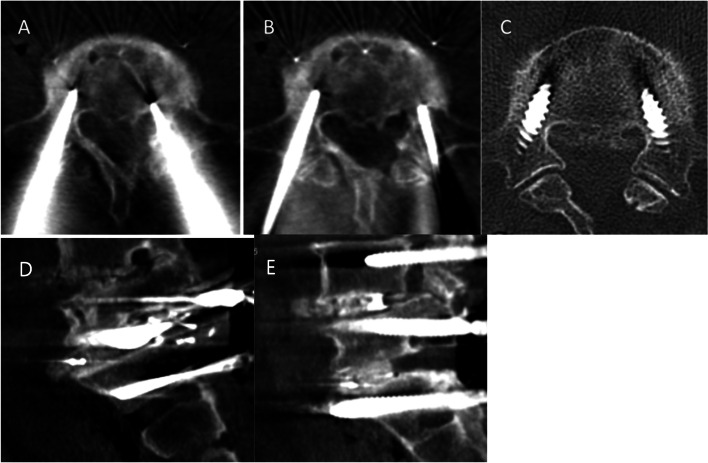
Examples of intraoperative computerized tomography (CT) images. A Initial intraoperative image demonstrating a medial misplacement of guiding needle in the left L5 pedicle. B Second intraoperative scan image showing the redirected needle properly located in the pedicle. C Postoperative CT image showing accurately placed pedicle screws. D, E Sagittal reconstruction images showing accurately placed needles (D) and screws (E)
